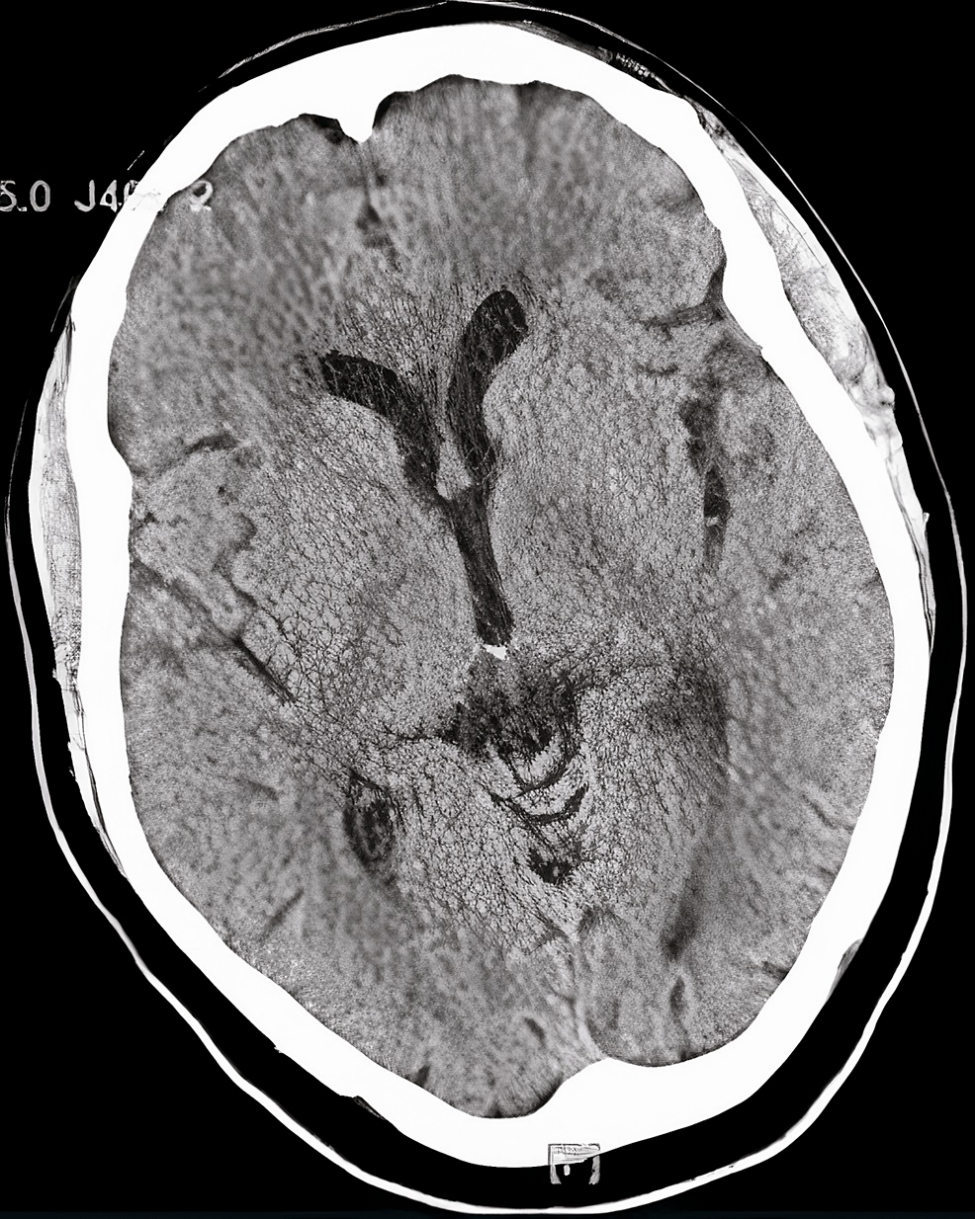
CT scan of the brain showing left temporal lobe volume loss.
